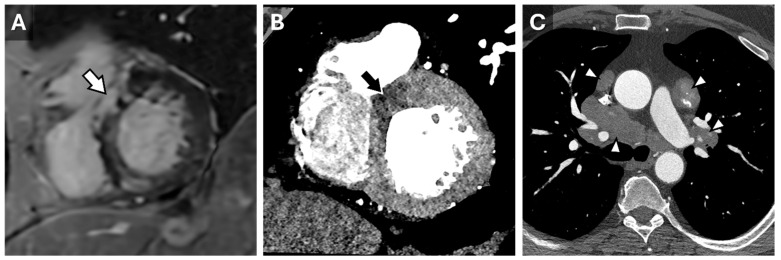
Sarcoidosis in CT Cardiac Imaging. CMR short-axis delayed-enhanced inversion recovery image through the LV base (A) from a patient with complete heart block and sarcoidosis with myocardial involvement. There is nonvascular delayed enhancement in the basilar septum (arrow). A contrast-enhanced cardiac CT from the same patient demonstrates a correlating area of decreased myocardial perfusion ((B), arrow). Axial reconstruction from the same CT (C) demonstrates partially calcified mediastinal and hilar lymphadenopathy (arrowheads) consistent with adenopathy in the setting of sarcoidosis.
